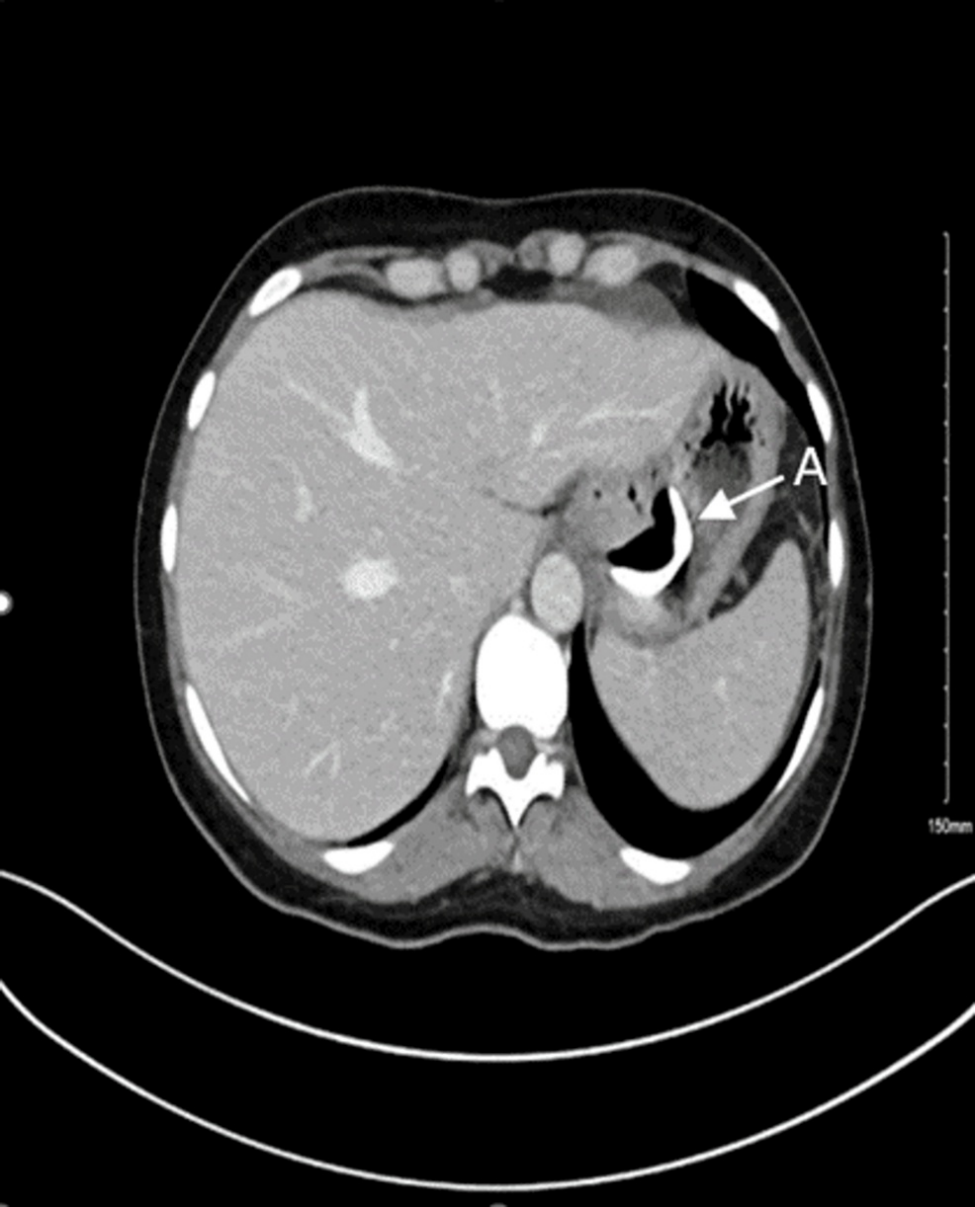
Computed tomography with contrast of the abdomen and pelvis showing findings of band eroding into the stomach (arrow A).
